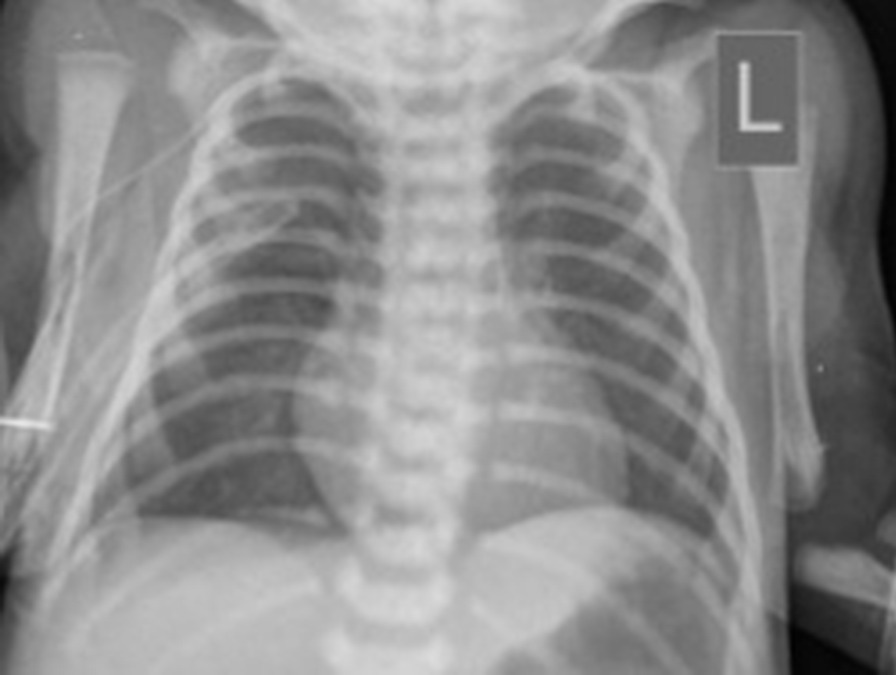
Chest radiograph of the newborn obtained on the fifth day of life
